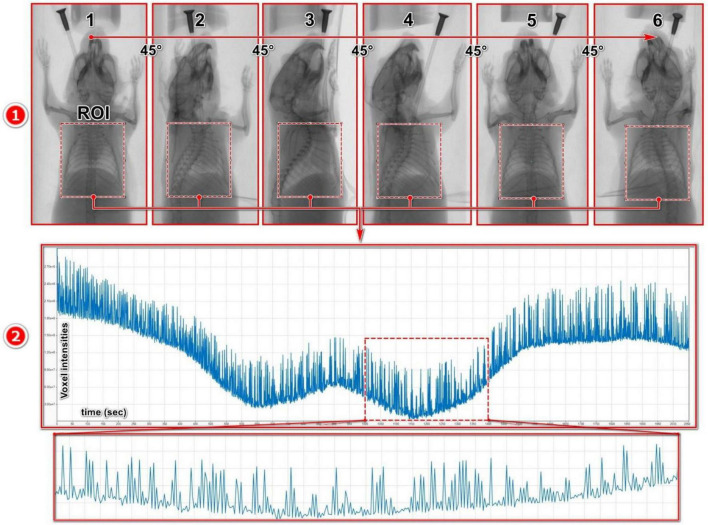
Breathing signal acquisition from projection images: (1) Multiple ROIs with benchmark angular positions separated by 45° rotation steps were manually placed on the lungs, encompassing the entire lung and diaphragm. (2) The exported intensity curve, derived from the signal acquisition process, represents the average pixel intensity within the ROIs across all X-ray projections within the associated timeframe.
